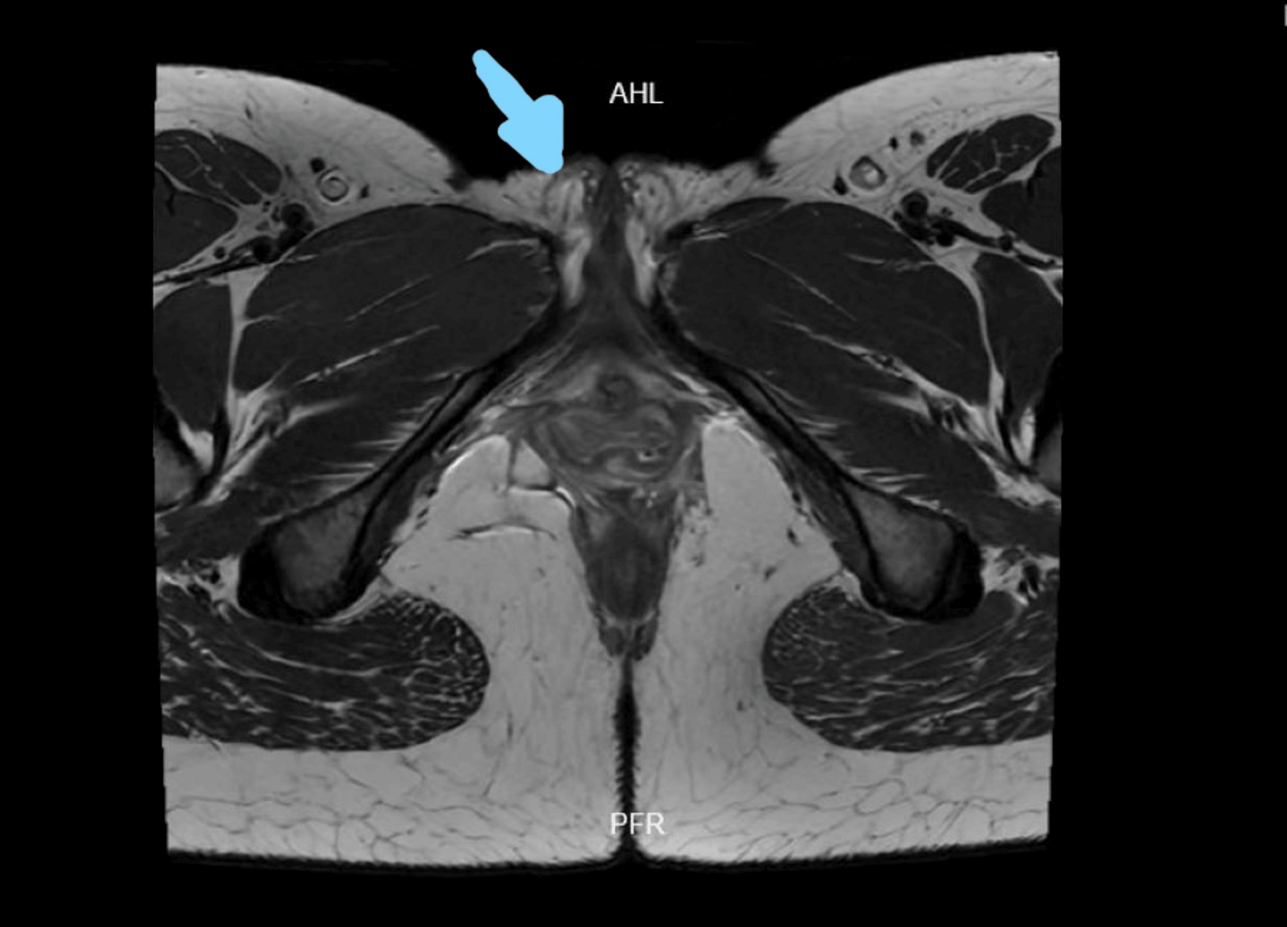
Nuclear magnetic resonance imaging of the pelvis without contrast enhancement (T2) The image shows a tumor lesion, with iso-, hypo-, and hyperintense areas measuring 2.3 cm in diameter, at the perineal level toward the perineal body topography, extending toward the right labia majora (blue arrow).
